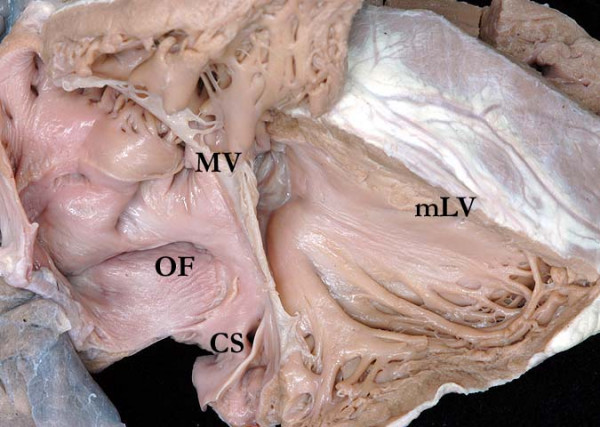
This dissection is made to replicate the parasternal long echocardiographic projection. It shows the coronary sinus (CS) opening to the right-sided morphologically right atrium, which connects to the morphologically left ventricle (mLV) through the mitral valve (MV). The oval fossa (OF) is clearly seen in the atrial septum
